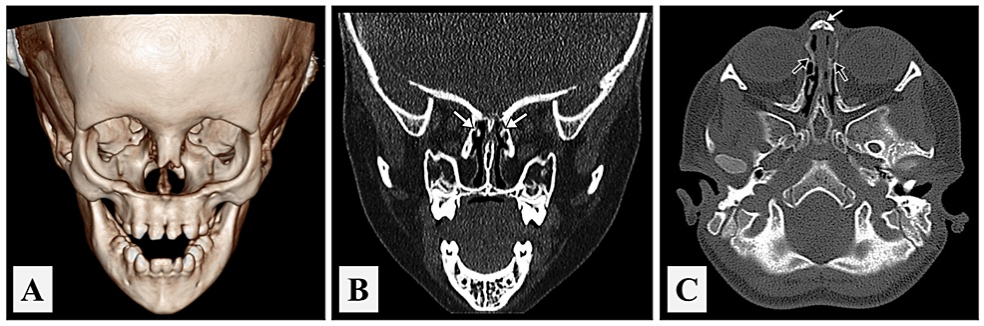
A computed tomography of a child with Johanson-Blizzard syndrome. (A) A three-dimensional computed tomography scan of facial bones; (B) a coronal computed tomography scan of the nose and paranasal sinuses showing aplasia of maxillary sinuses, small middle turbinates (arrows), and absent inferior turbinates; (C) an axial computed tomography scan of the nose and paranasal sinuses showing short nasal bones (white arrow), absent frontal processes of maxillary bones, and loss of bony boundaries of nasolacrimal ducts (black arrows).
